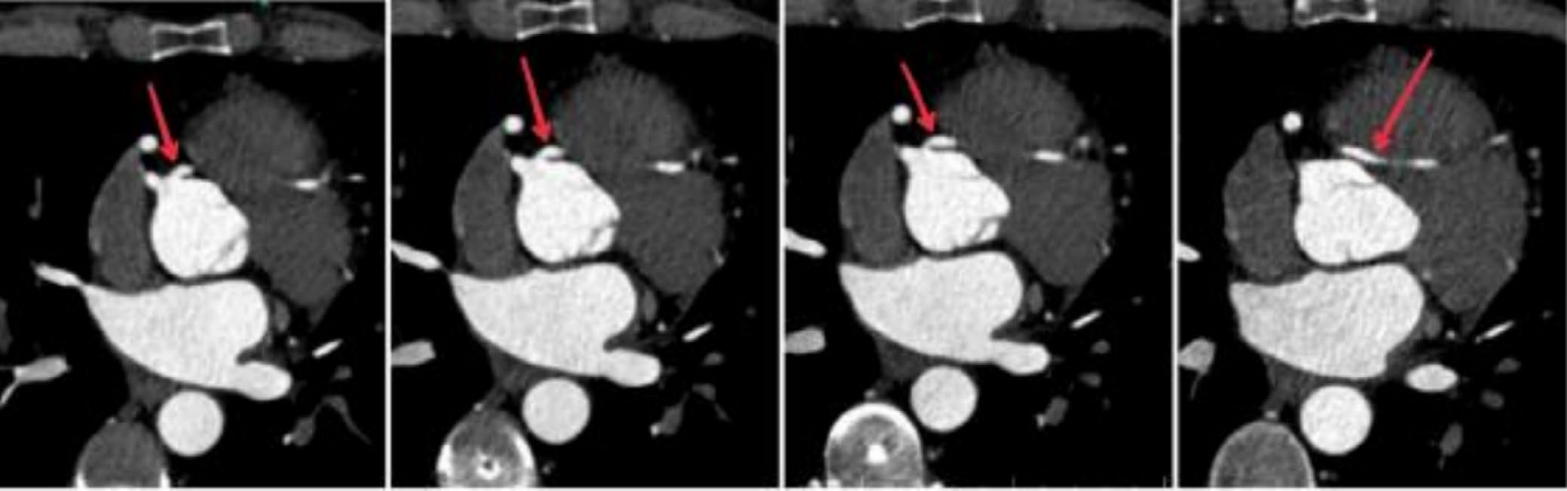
Coronary computed tomography angiography: It shows the origin of the left main coronary artery and its transseptal course.
